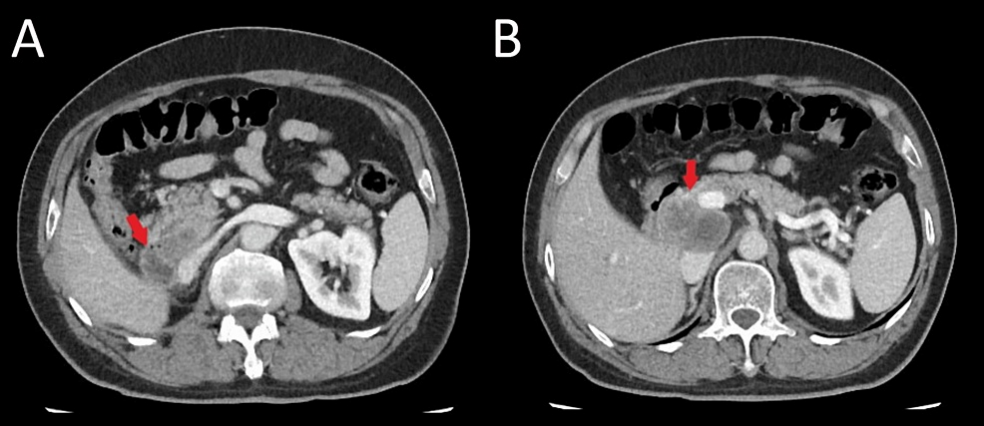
Abdominal CT scan The abdominal CT scan shows a necrotic mass compressing the common bile duct (CBD), with no other pathologic finding elsewhere.
